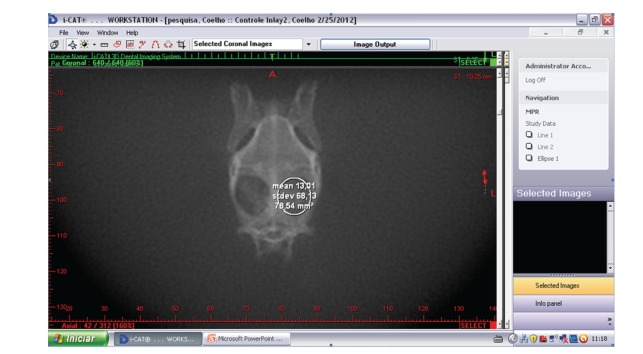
CT view after the measurement of a residual defect area.
